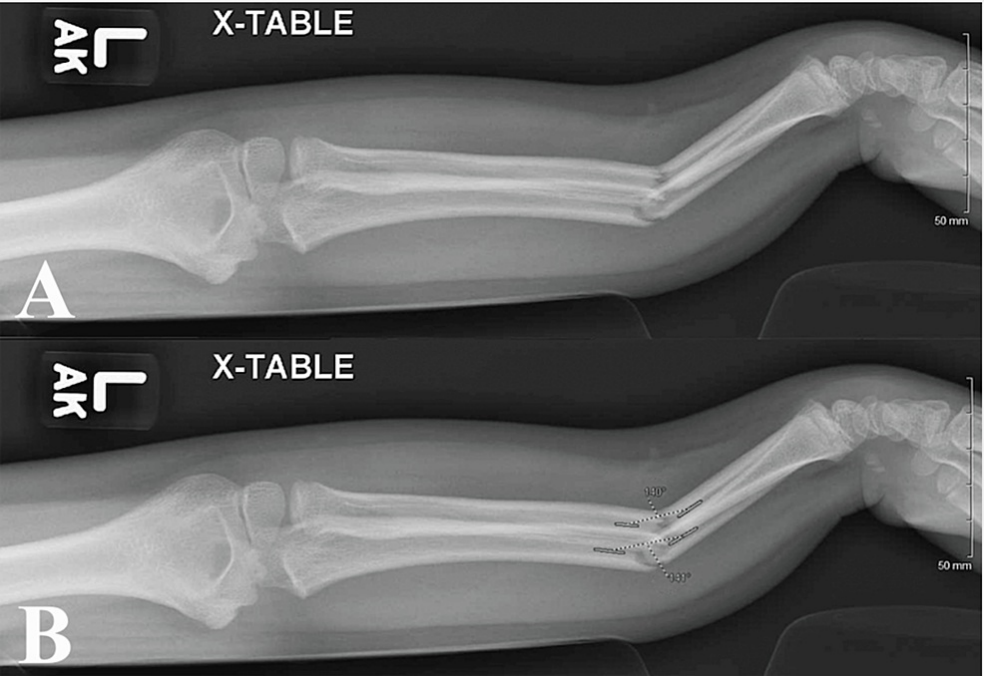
Pre-reduction radiograph in lateral view with measurements unlabeled (A) and labeled (B). Gray markings measure the angle of deformity.
